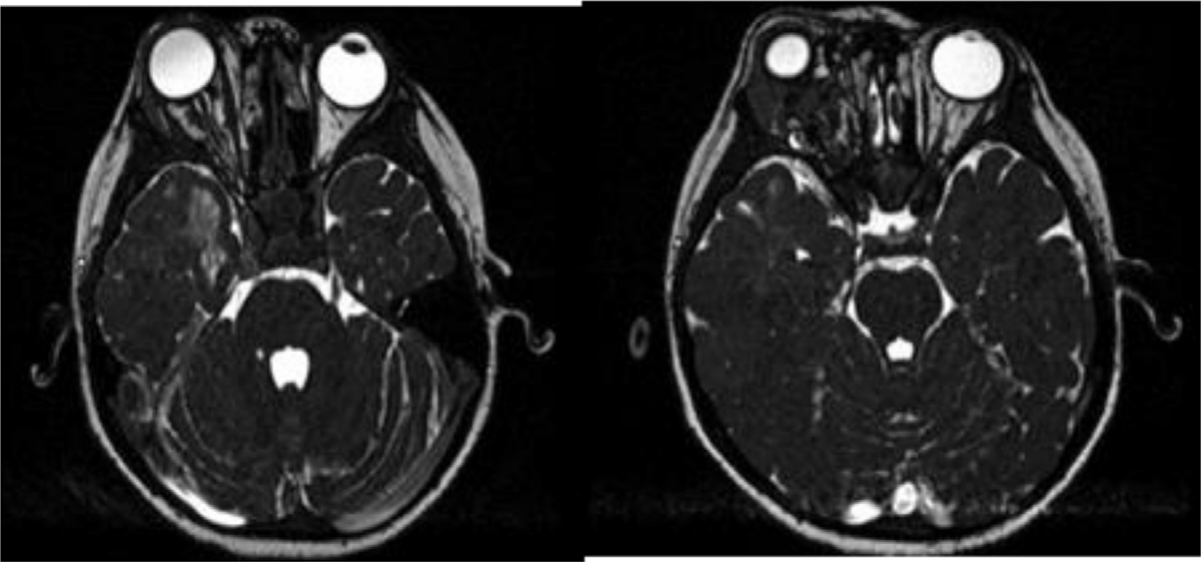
MRI postsclerotherapy showed a smaller inhomogeneous lesion in the right retrobulbar with the distance between the anterior margin of the right ocular bulb and the right interzygomatic line reduced to 2.12 cm.
